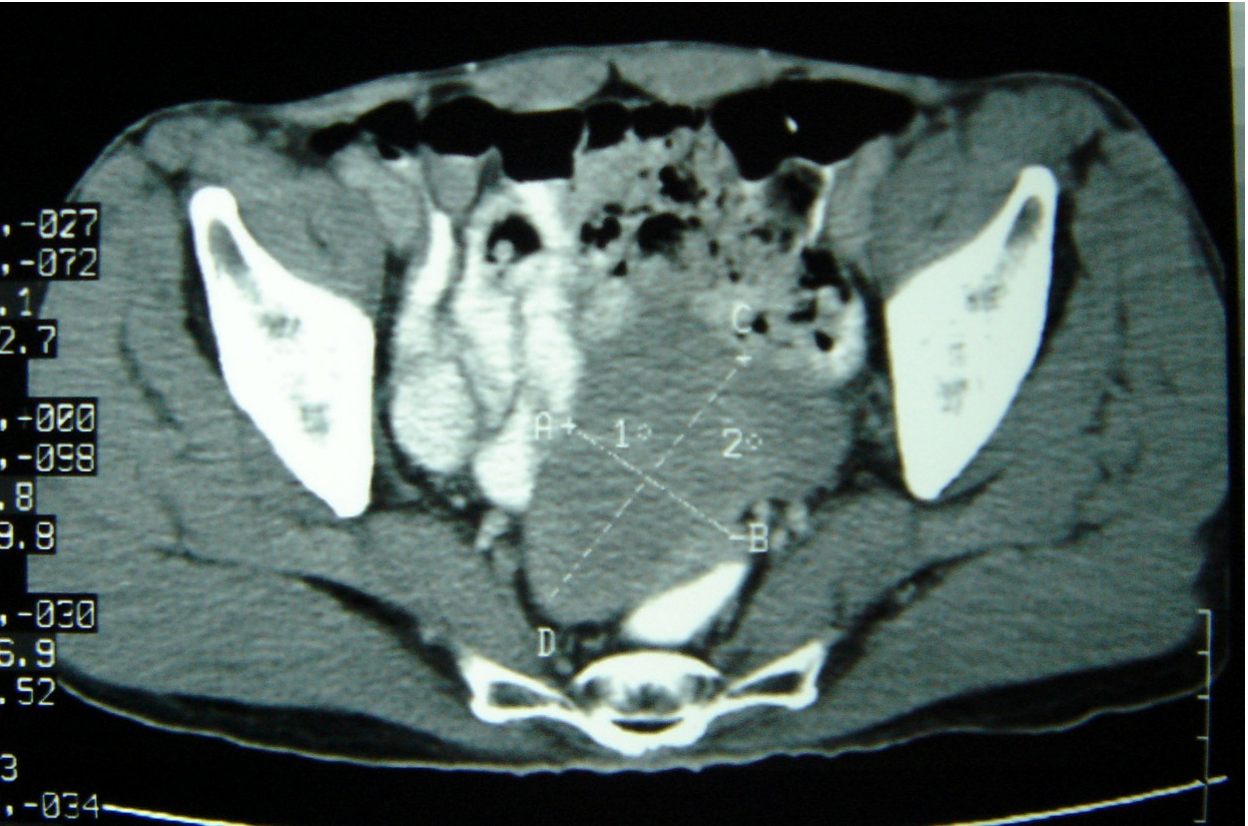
Preoperative CT scan showing pelvic mass; Contrast enhanced CT scan showing lobulated mass lesion in pelvis compressing anterior wall of sigmoid colon and located posteriosuperior to the urinary bladder.
